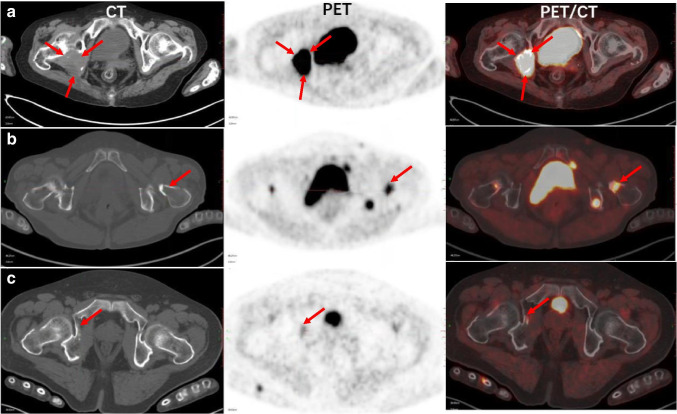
Representative [^18^F]AlF-NOTA-FAPI-04 PET/CT scans of bone metastasis in patients with SCC (a SUV_max_ = 26.27), ADC (b SUV_max_ = 13.2) and SCLC (c SUV_max_ = 6.24). Red arrow points to bone metastasis
